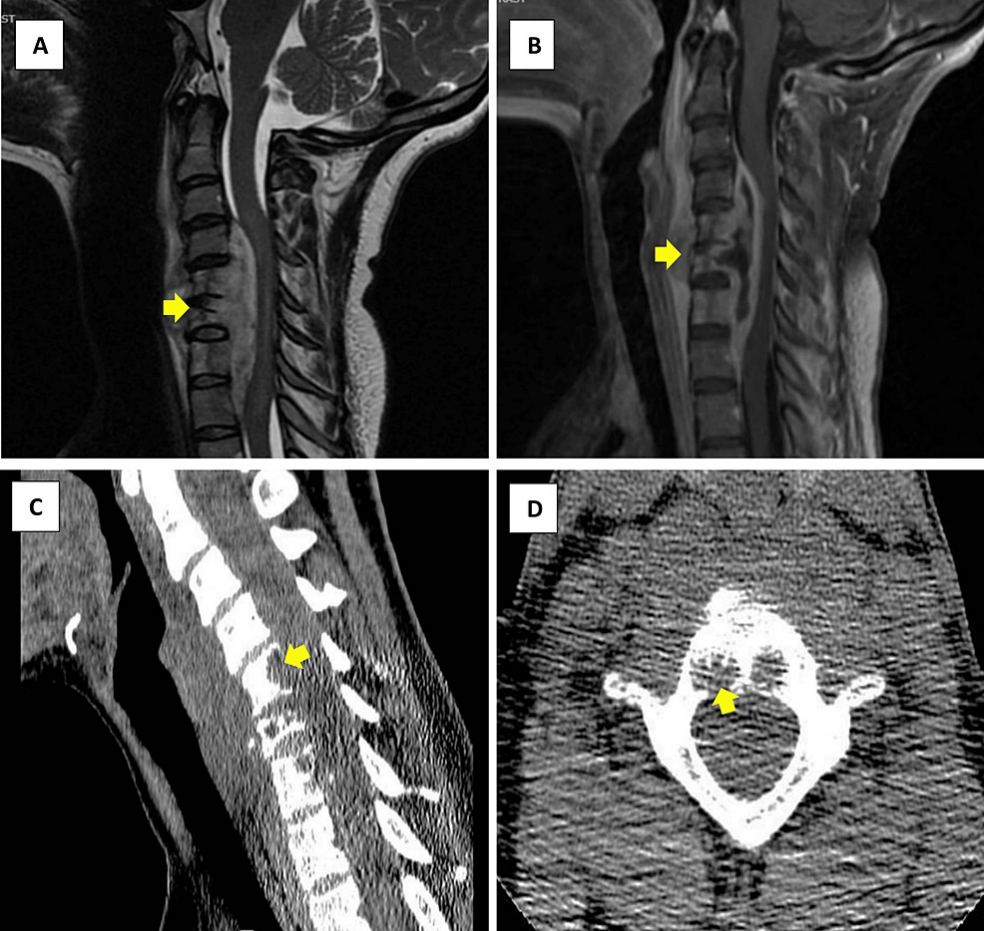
MRI and CT images of the cervical spine Images A and B show peripherally enhancing mass-like lesions (arrows) involving C5, C6, and C7 with significant spinal canal stenosis on MRI of the cervical spine. Images C and D show sagittal and axial cuts, respectively, of the mass-like lesions on the CT scan of the neck.
